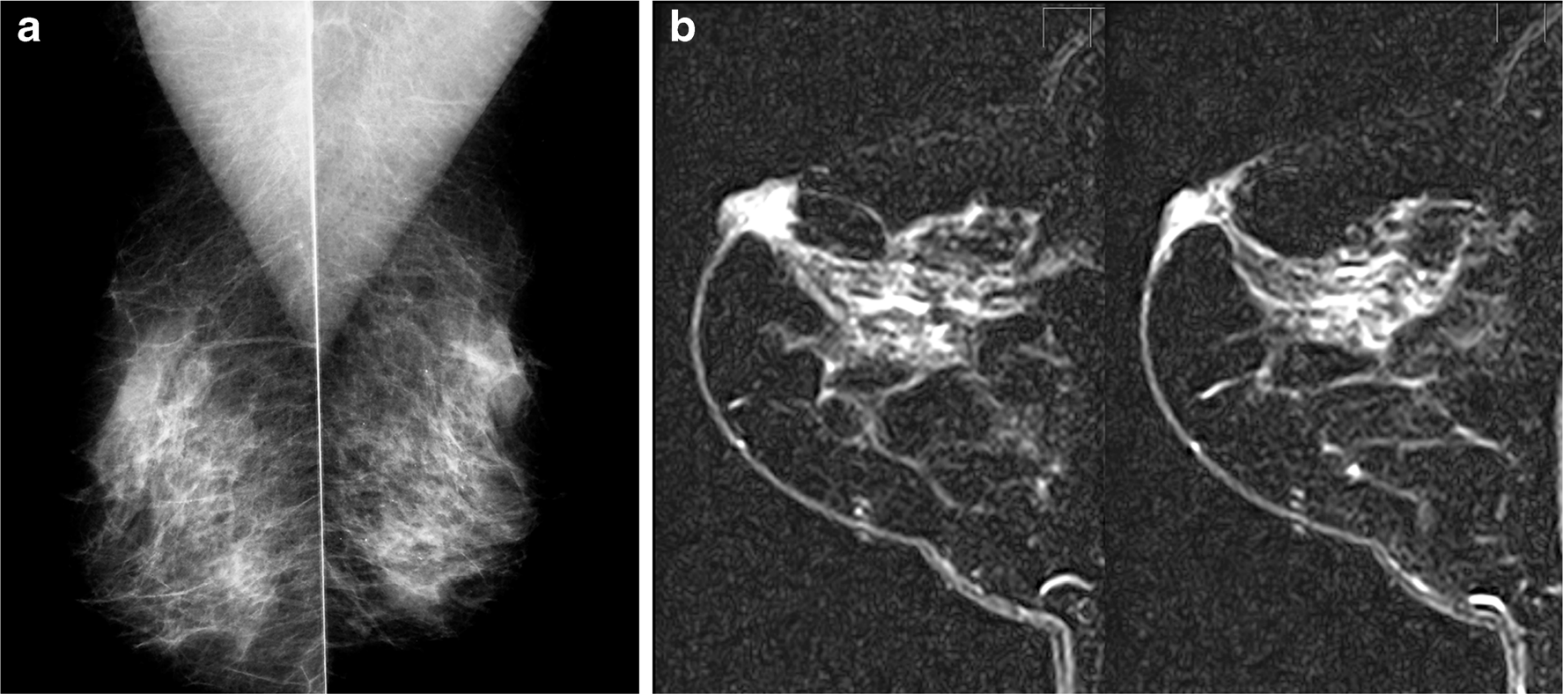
A 47-year-old patient presented clinically with minimal thickening in thenipple areolar region in the left breast. Mammogram (MLO view) of both breasts (a) was normal, while MRI of the left breast (b) showed enhancing thickening in nipple areolar region with no obvious underlying mass
